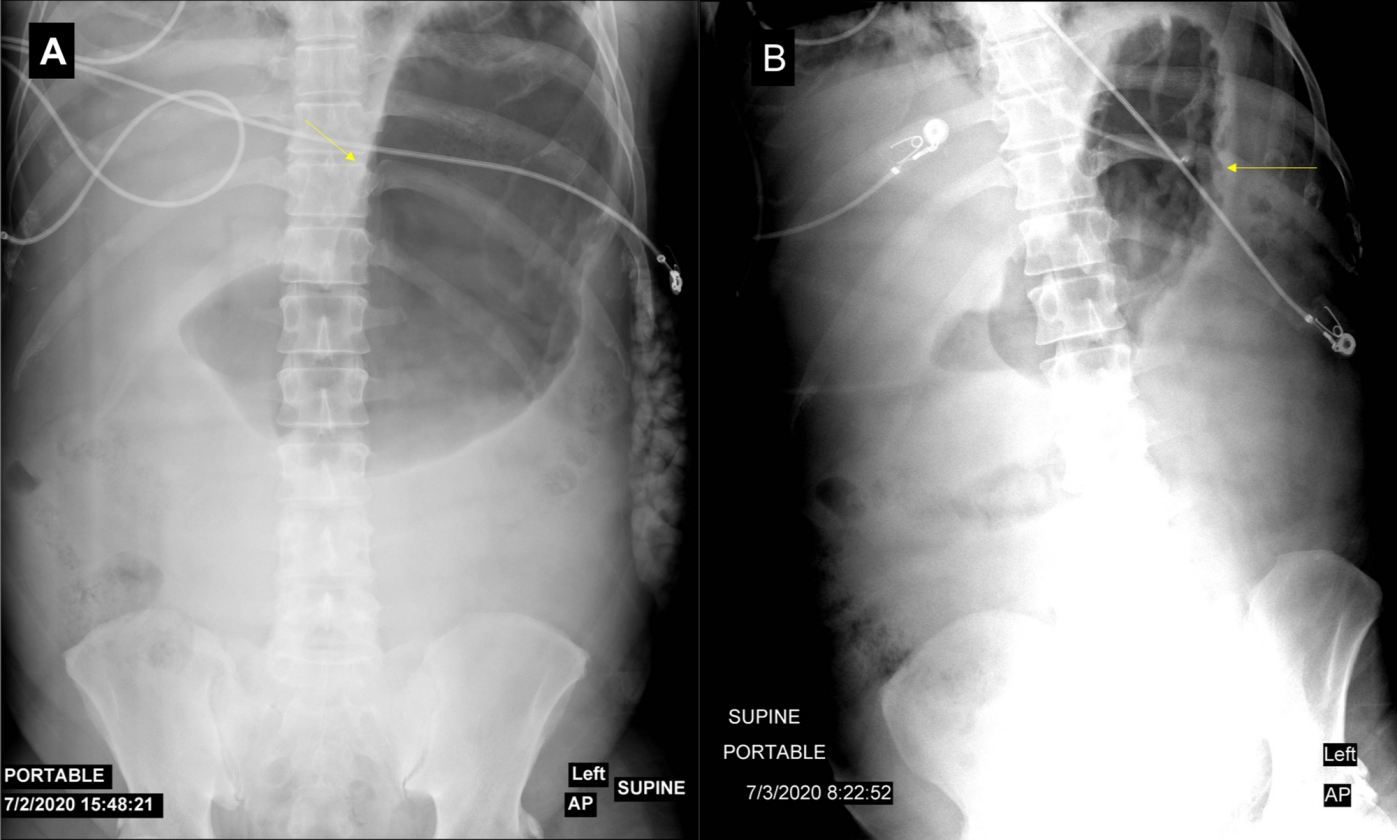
Kidney, ureter, and bladder (KUB) X-ray A: the image shows gastric distention (yellow arrow). B: the image shows gastric decompression after the insertion of the nasogastric tube (yellow arrow)
